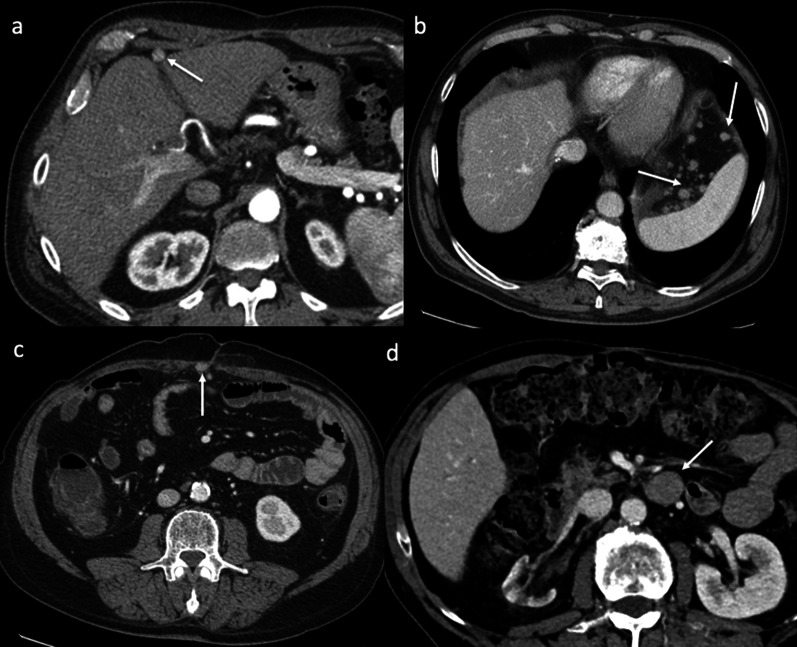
Peritoneal recurrence. Axial contrast-enhanced CT images (a–d) show different patients with peritoneal HCC recurrence (arrows), characterized by single or multiple discrete masses located in the peritoneal cavity or omentum. These intraperitoneal masses are often hypervascular on the arterial phase, such as the primary hepatic mass
